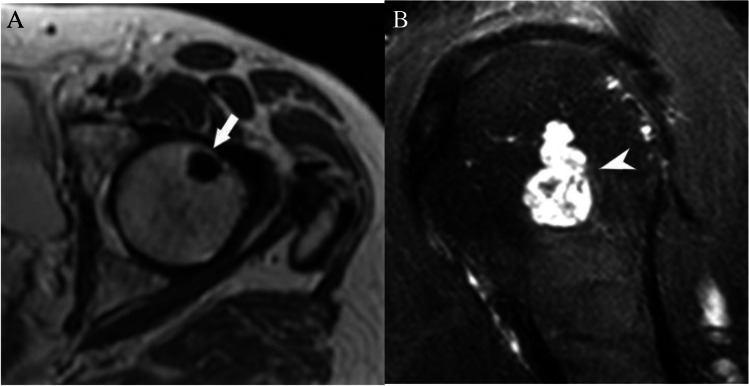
MRI lesions based on T2 signal intensity. A Axial T2-weighted MRI image of the hip shows a uniformly hypointense lesion (arrow) in the femoral head consistent with an enostosis. B Sagittal T2-weighted fat-suppressed MRI images show a lobulated hyperintense lesion in the humeral head consistent with a low-grade cartilage lesion/enchondroma
